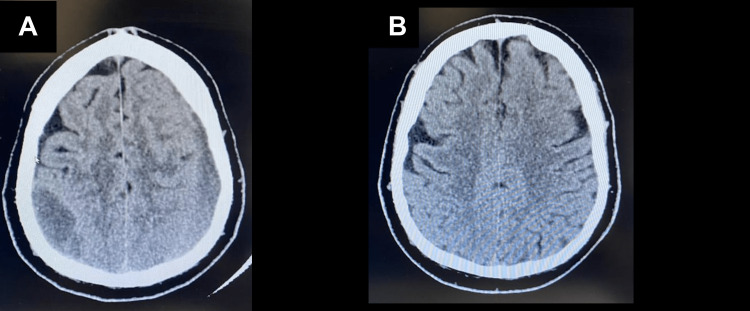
Axial CT scan of the brain: (A) bilateral parietal hypodense fluid collection and (B) bilateral epidural hematoma resolution. CT: computed tomography
